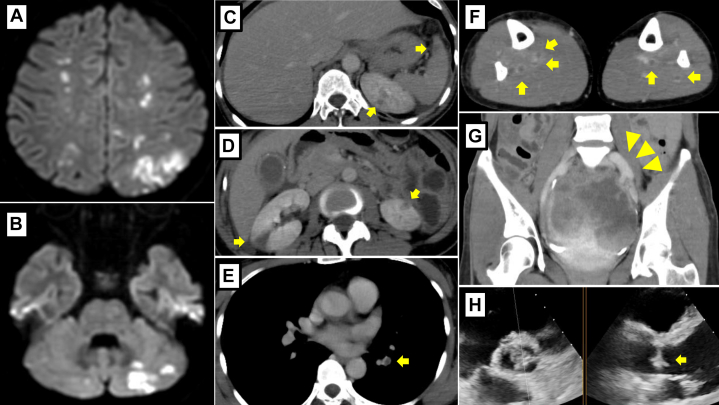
Initial Diagnostic Findings (A and B) Multiple cerebral infarctions identified on brain MRI. (C and D) Splenic infarction and multiple renal infarctions observed on contrast-enhanced CT (arrows). (E) Pulmonary embolism in the lower lobe of the left lung (arrow). (F) Multiple deep vein thromboses (arrows). (G) A large contrast-enhanced pelvic mass (arrowheads). (H) Mobile vegetations on aortic valve detected by TTE (arrow). CT = computed tomography; MRI = magnetic resonance imaging; TTE = transthoracic echocardiography.
